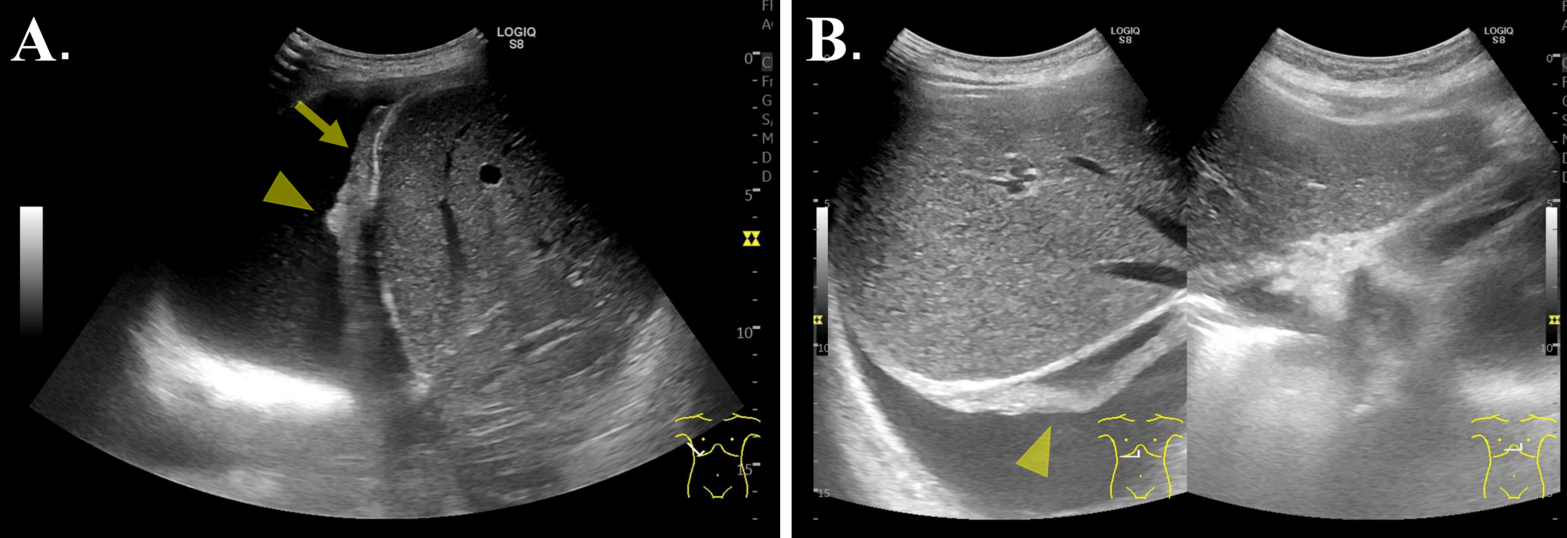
(A and B) Right pleural effusion accompanied by pleural thickening (arrow) and subpleural nodules (arrowhead) identified on lung ultrasound (with convex probe).
